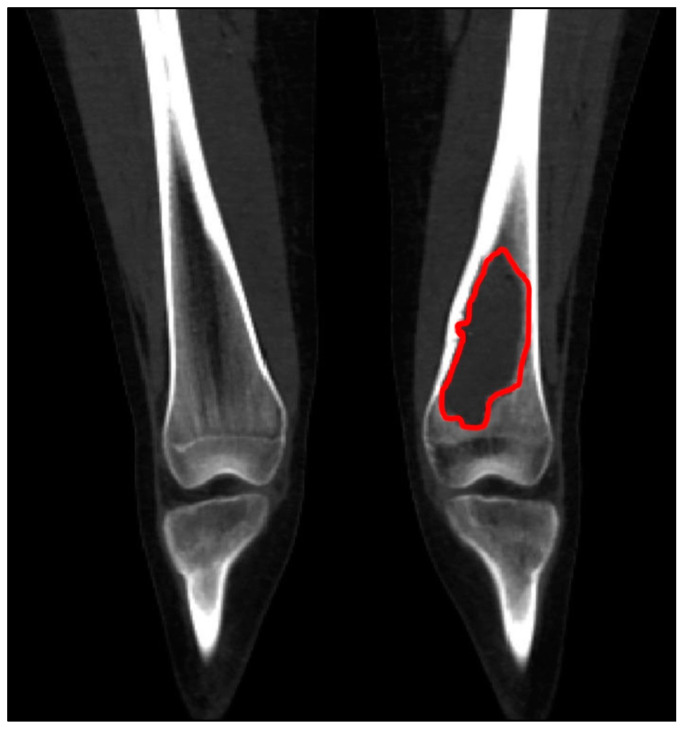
An example of an MRI demarcating the tumor margins (red line) superimposed over a CT scan demarcating the bone anatomy.
